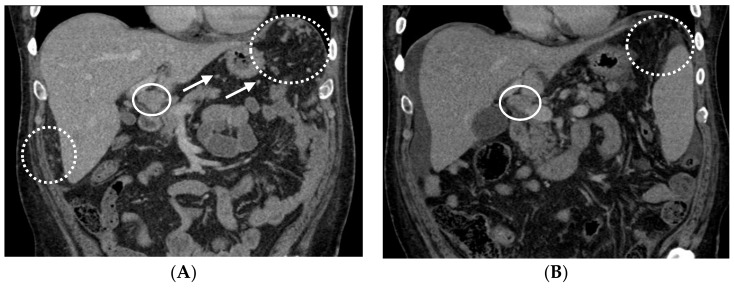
Following cycle 3 and cycle 6 with capecitabine, oxaliplatin, and bevacizumab (CAPOX-B), the CT scans showed tumor response. (A) Following cycle 3 with CAPOX-B, the coronal contrast-enhanced CT scans demonstrate innumerable left sub-diaphragmatic and peri-hepatic omental nodules (dashed circles) along with peri-gastric nodules (several annotated by arrows) consistent with omental carcinomatosis and mesenteric metastases. Peri-portal lymphadenopathy can be observed in the solid circle. (B) Following cycle 6 with CAPOX-B, the coronal contrast-enhanced CT scans demonstrate a decrease in omental metastases (dashed circle) and peri-portal lymphadenopathy (solid circle), and a small amount of peri-hepatic ascites.
